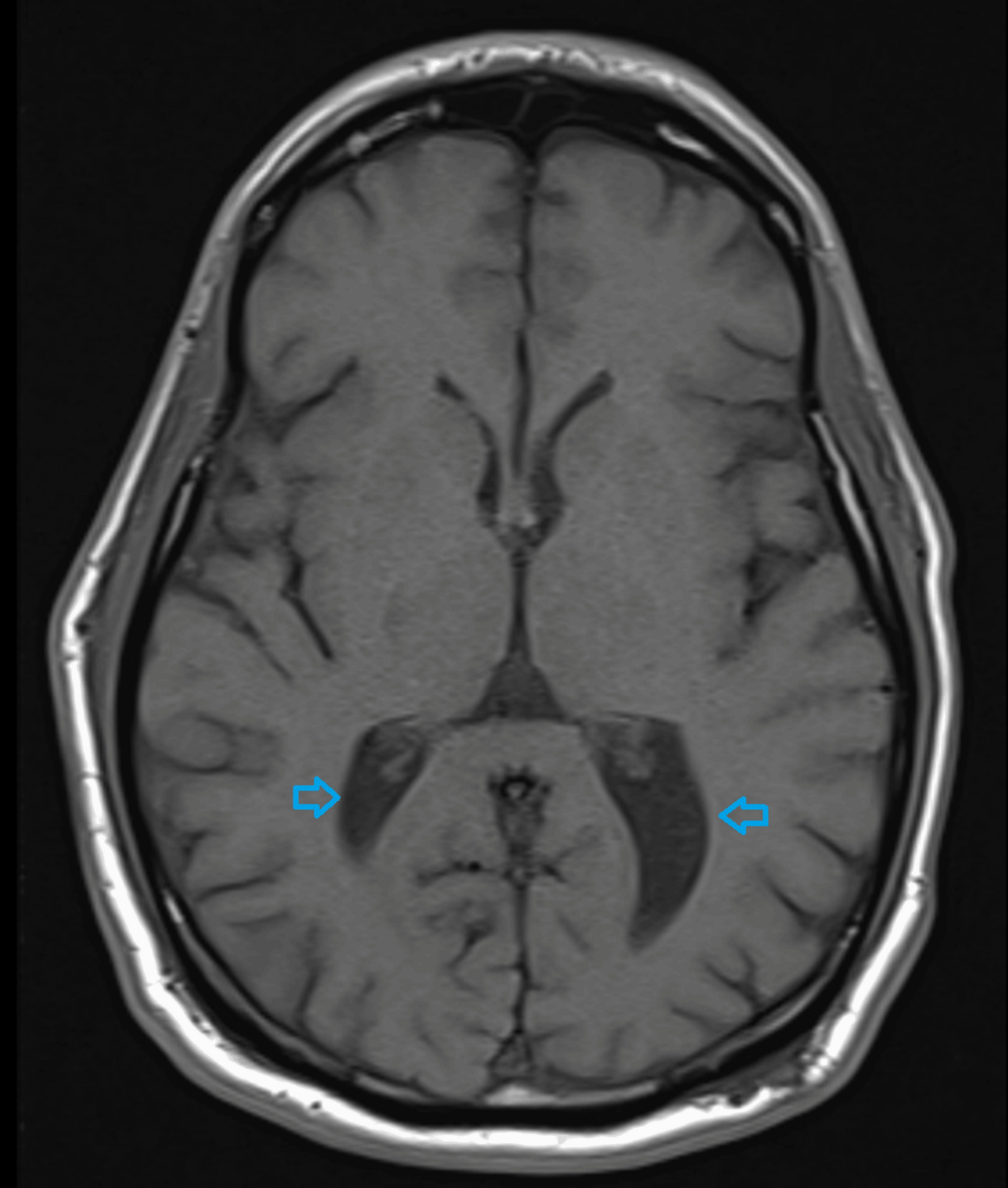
Axial brain MRI showing the mild enlargement of the occipital horn of the lateral ventricles (blue arrows)
